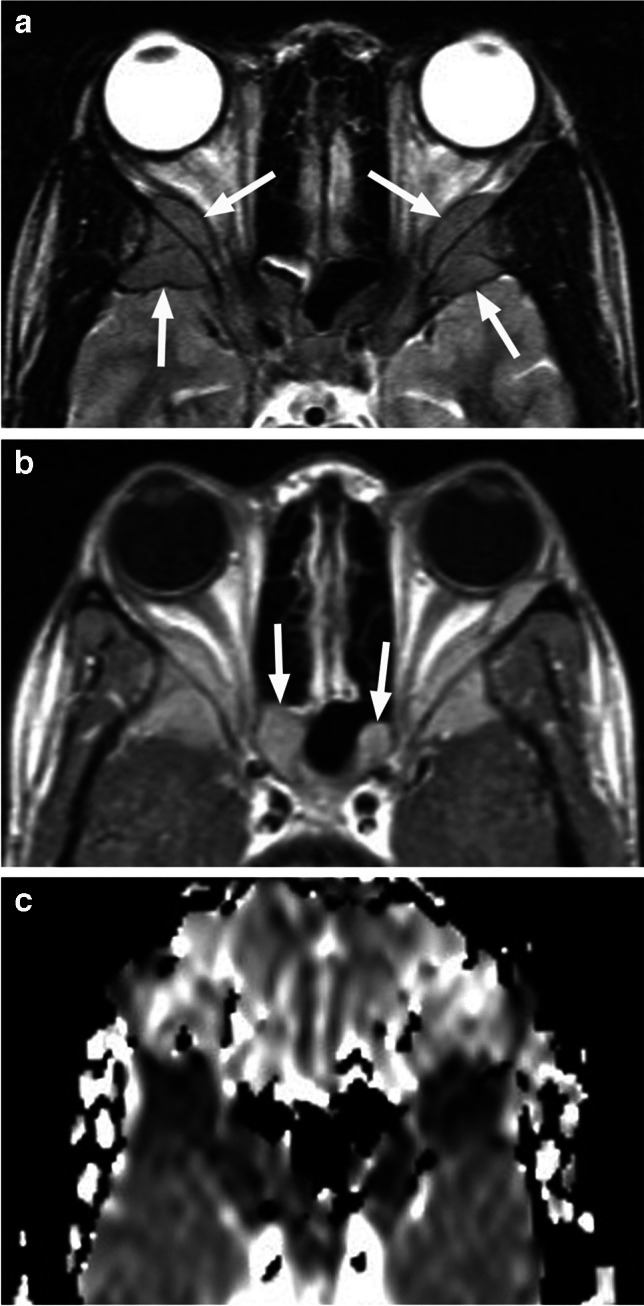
Axial T2-weighted image of the orbits (a) shows bilateral homogenous mildly hypointense masses (arrows) centered at the posterior lateral orbital walls/sphenoid wings. There are preserved central hypointense lines in the cortical bone location. Corresponding postcontrast T1-weighted image (b) demonstrates mild homogenous enhancement of the lesions, which also extend into the sphenoid sinuses (arrows). Axial ADC map at a similar level (c) reveals dark signal of the masses, representing very low diffusivity. Acute myeloid leukemia (AML) was diagnosed in this patient presenting with orbital myeloid sarcoma
